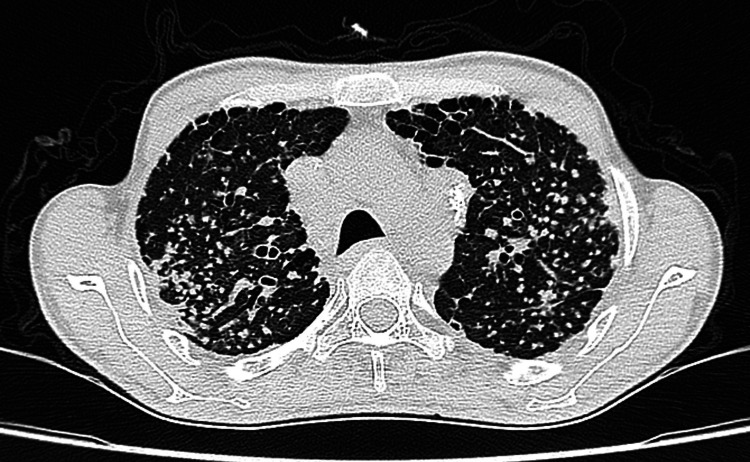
Chest HRCT showing numerous well-defined small nodules noted found in centrilobular distribution, predominately in the upper lobe in both lungs HRCT: high-resolution computed tomography
